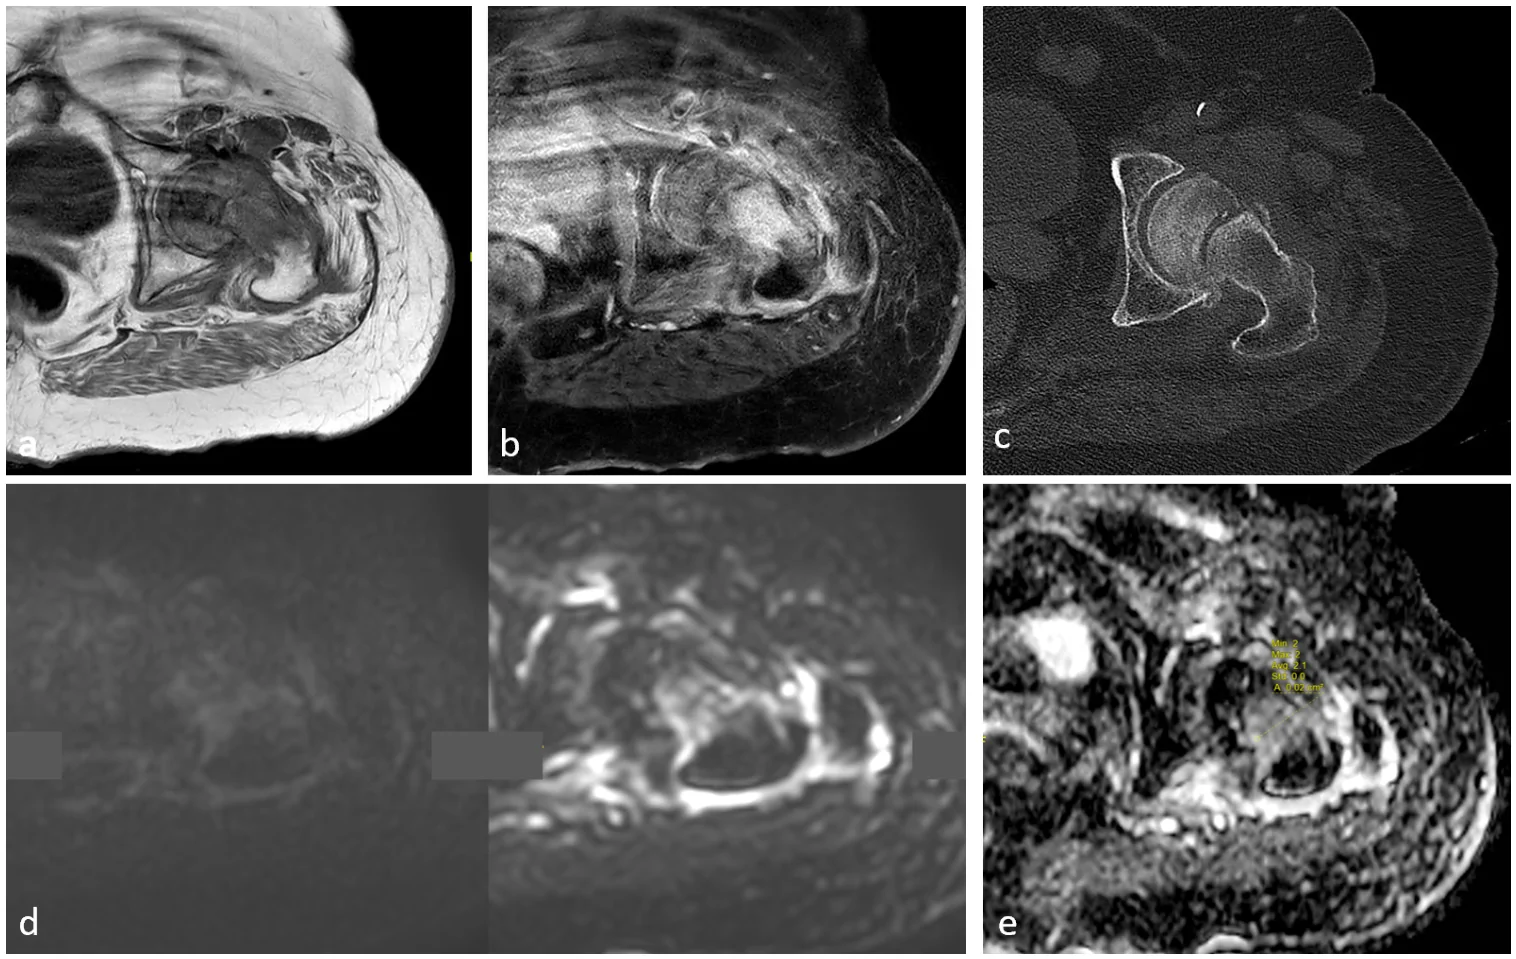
Axial MRI and CT of an elderly patient with trivial trauma, performed to exclude a pathological fracture of the femoral neck. Reactive vasogenic oedema surrounding the fracture site demonstrates T1-weighted hypointensity (a) with high signal on both DWI and the ADC map (d,e), reflecting T2 shine-through rather than true restricted diffusion. The absence of focal restricted diffusion supports a benign fracture when taken together with the morphology, clinical history and surgical fixation; some malignant lesions may show little or no restriction. Notably, significant reactive post-contrast enhancement is present at the fracture site (b), which may obscure or mimic pathological enhancement from an underlying lesion. CT (c) showed fracture without underlying lytic lesion.
